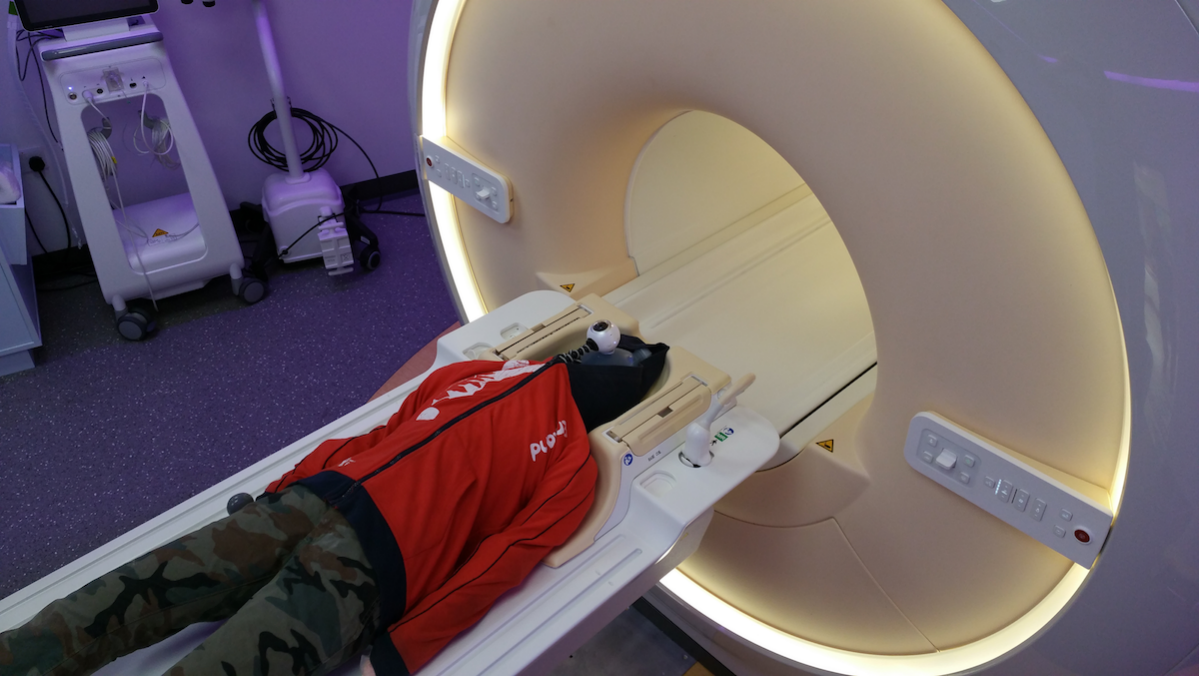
Setup for filming 360 degree video within an operating magnetic resonance imaging scanner.
